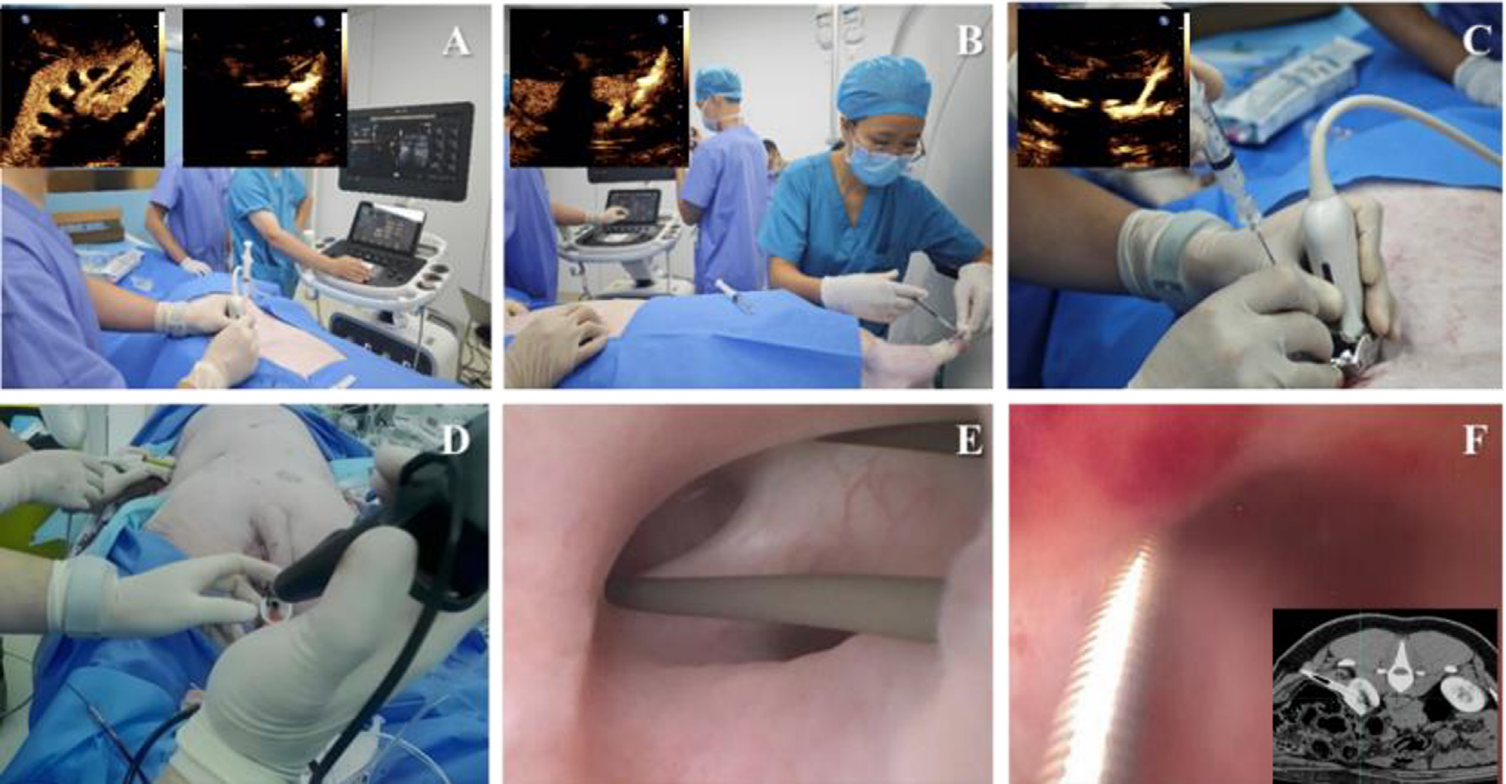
Stepwise procedure for percutaneous access to the renal collecting system in an *in vivo* model. (A) The contrast-filled needle tip was advanced to the renal capsule. (B) Following intravenous injection of 0.5 ml contrast agent, vascular filling was observed and puncture was performed through relatively avascular areas. (C) Upon needle entry into the target calyx, diluted contrast agent (1:100) was injected through the needle to visualize the collecting system. (D and E) Guidewire placement was confirmed via both retrograde and antegrade ureteroscopy. (F) CTA confirmed successful establishment of the dilated tract. CTA = computed tomographic angiography.
